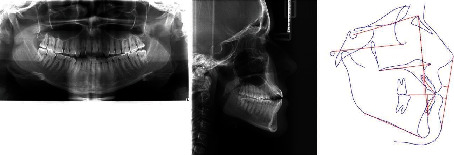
Initial panoramic and lateral cephalometric radiography.
